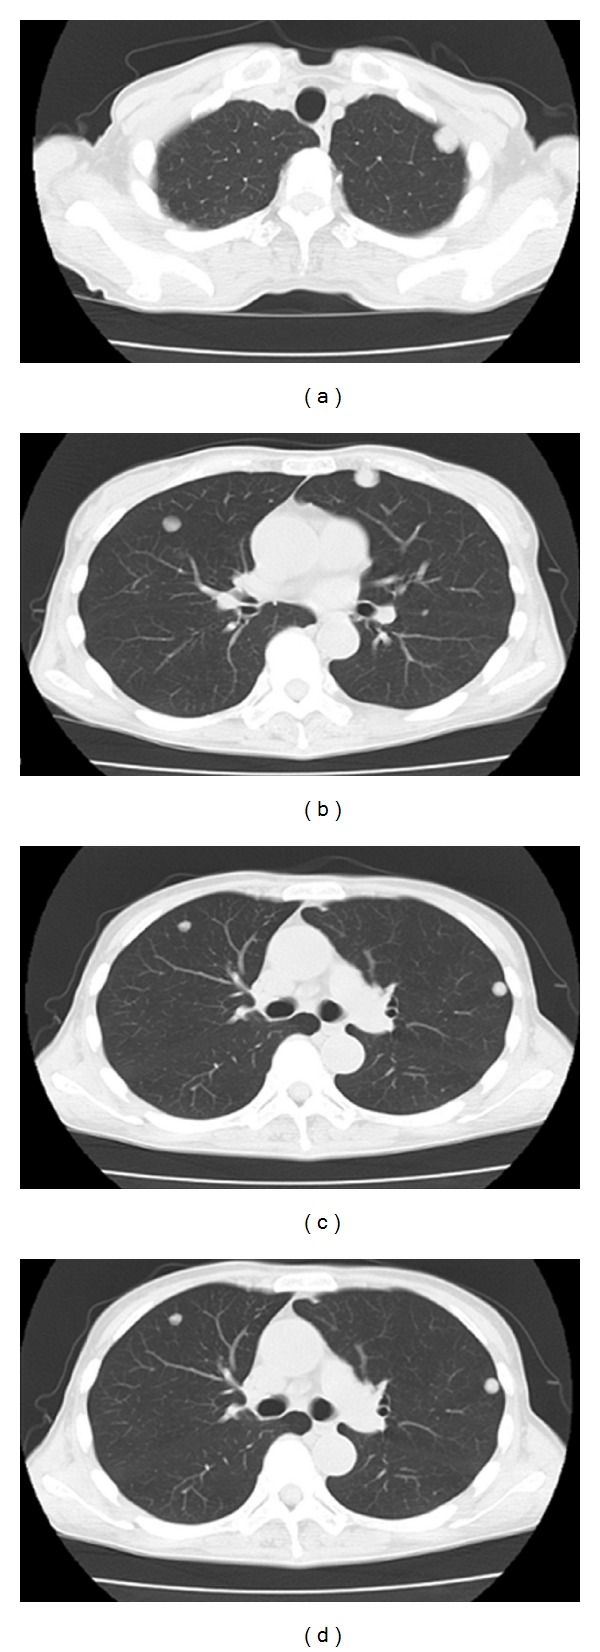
CT examination two months after CRS revealed rapid progression of multiple lung metastases.
